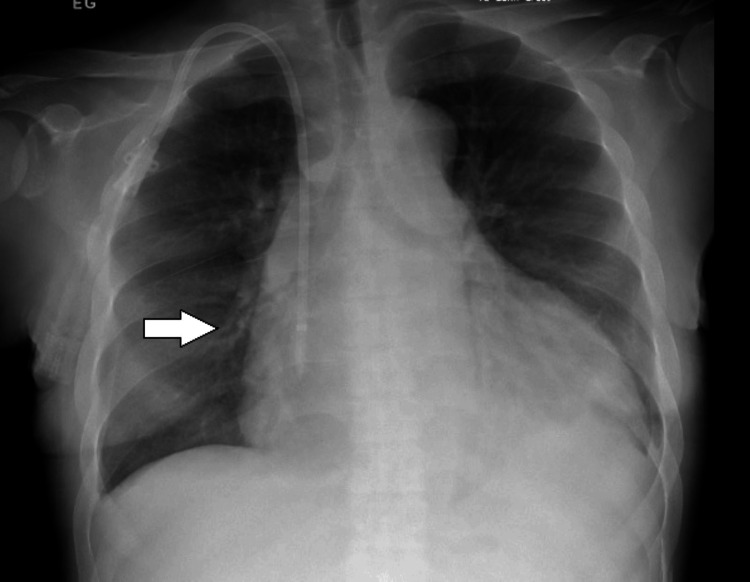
Chest X-ray showing vascular congestion (white arrow)
